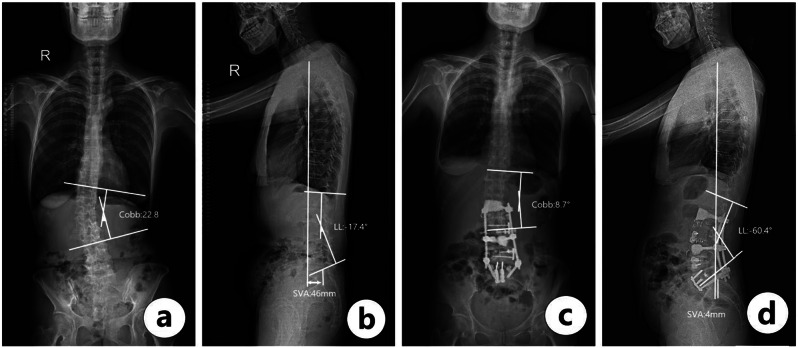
(a, b) Preparative frontal and lateral view radiographs (c, d) Postoperative frontal and lateral view radiographs
